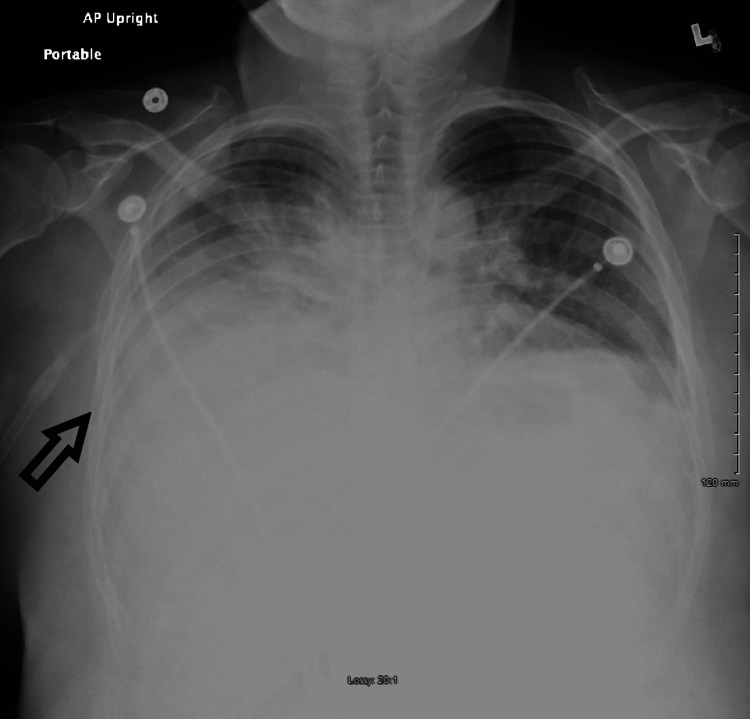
Chest X-ray showing bilateral pleural effusions with right worse than left (arrow).
